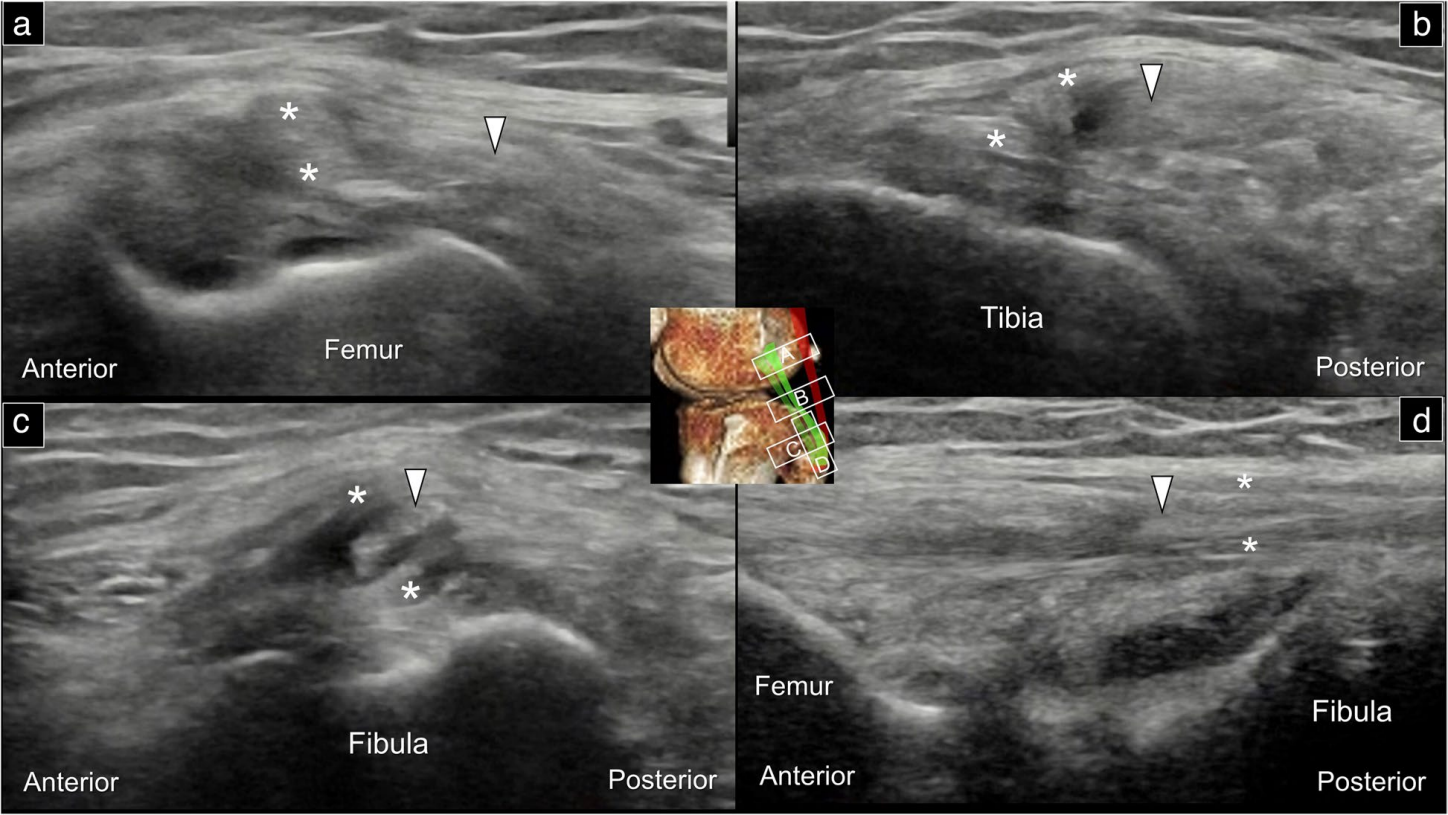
Ultrasound imaging of the bifid lateral collateral ligament (asterisk) from the origin (a) and middle part (b) to the insertion (c) in the short-axis and long-axis (d) views. White arrowheads: biceps femoris tendon. Please reference Supplemental Fig. 2 for the normal depiction of the biceps femoris tendon and its relationship with the lateral collateral ligament
